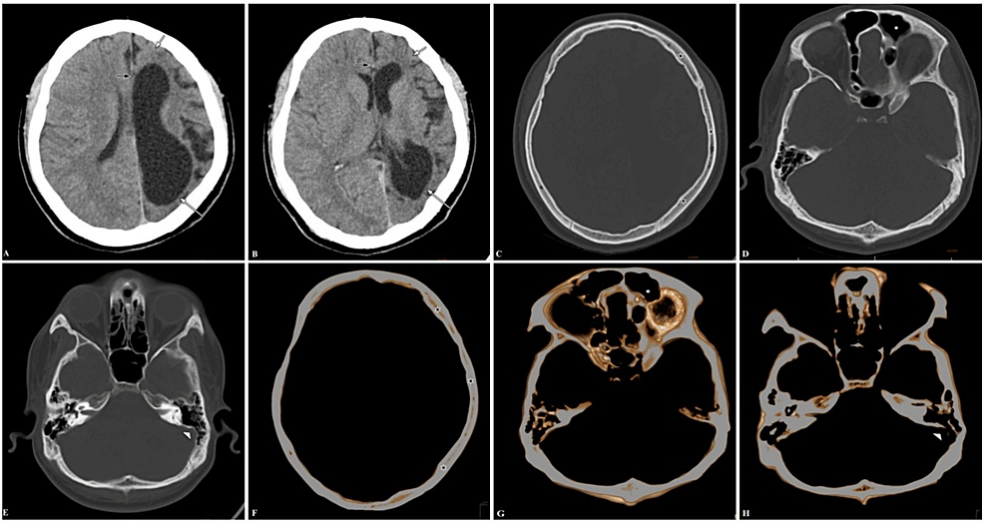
Case presentation 2 - CT brain Axial sections in the brain window (A, B) show left hemicerebral atrophy (short white arrow). There is ipsilateral lateral ventricle ex vacuo dilatation (long white arrow) and a minimal midline shift of falx cerebri to the ipsilateral side (short black arrow) at the corona radiata and Sylvian fissure level, respectively. Axial sections in the bone window (C, D, E) and 3D axial VR (F, G, H) show compensatory ipsilateral calvarial hypertrophy (black asterisks), ipsilateral frontal sinus hyperpneumatization (white asterisk), and ipsilateral mastoid air cells hyperpneumatization (small white arrowhead), respectively. CT: computed tomography; VR: volume rendering
